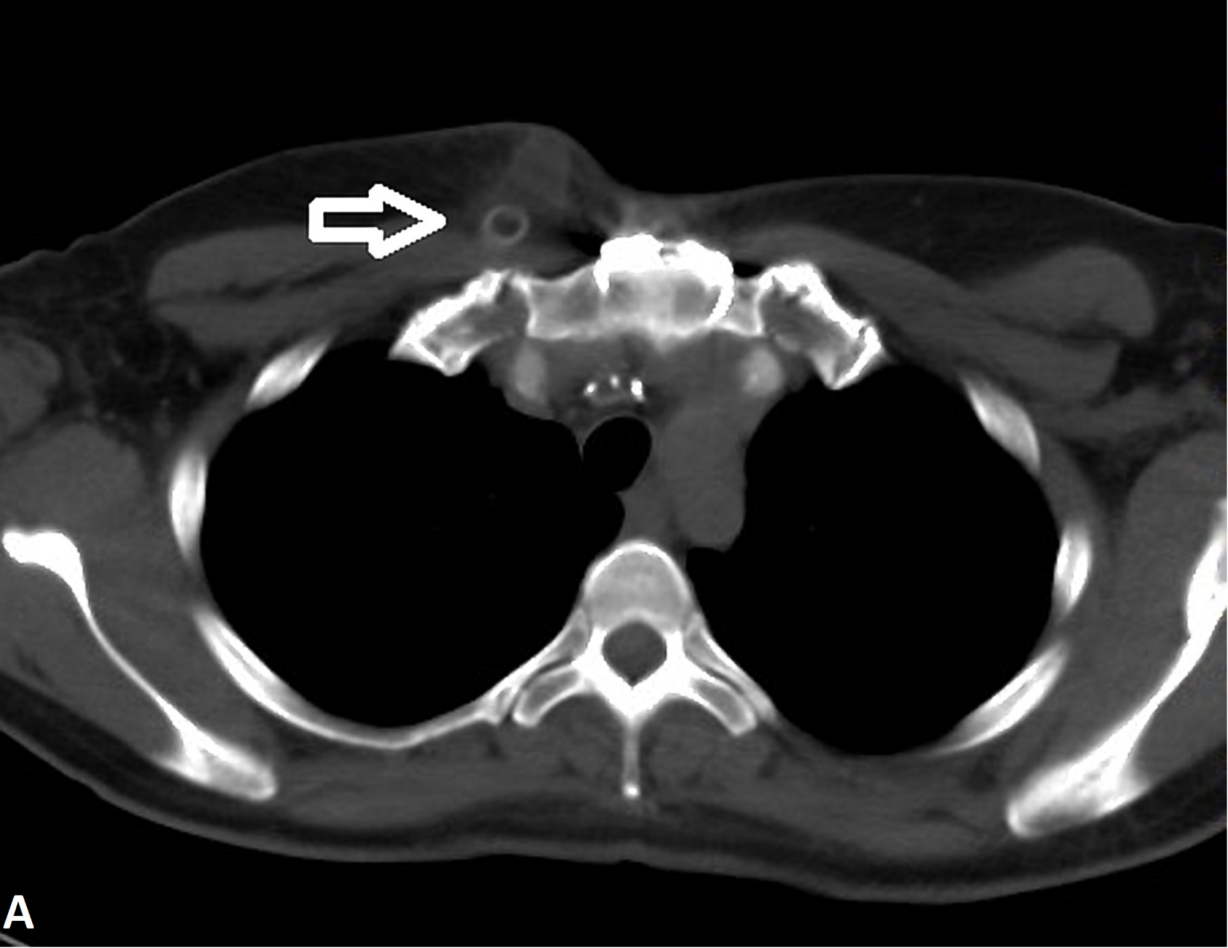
CT angiogram of the chest showing an area of fluid collection in the right paramedian subcutaneous anterior chest wall adjacent to the anterior aspect of the occluded aorto-axillary graft (arrow). This represented seroma or lymphocele. There is adjacent fat stranding, which may represent the scarring of cellulitis.
